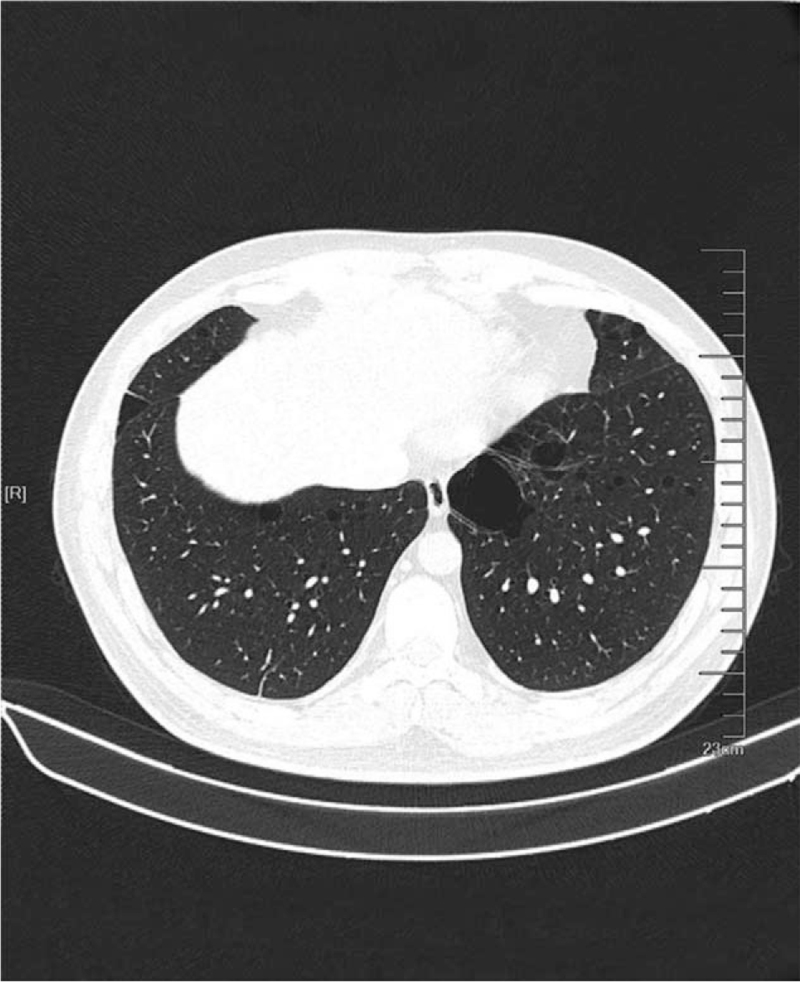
Plain Chest CT: multiple cystic low-density shadows in both lungs were irregular in size.
